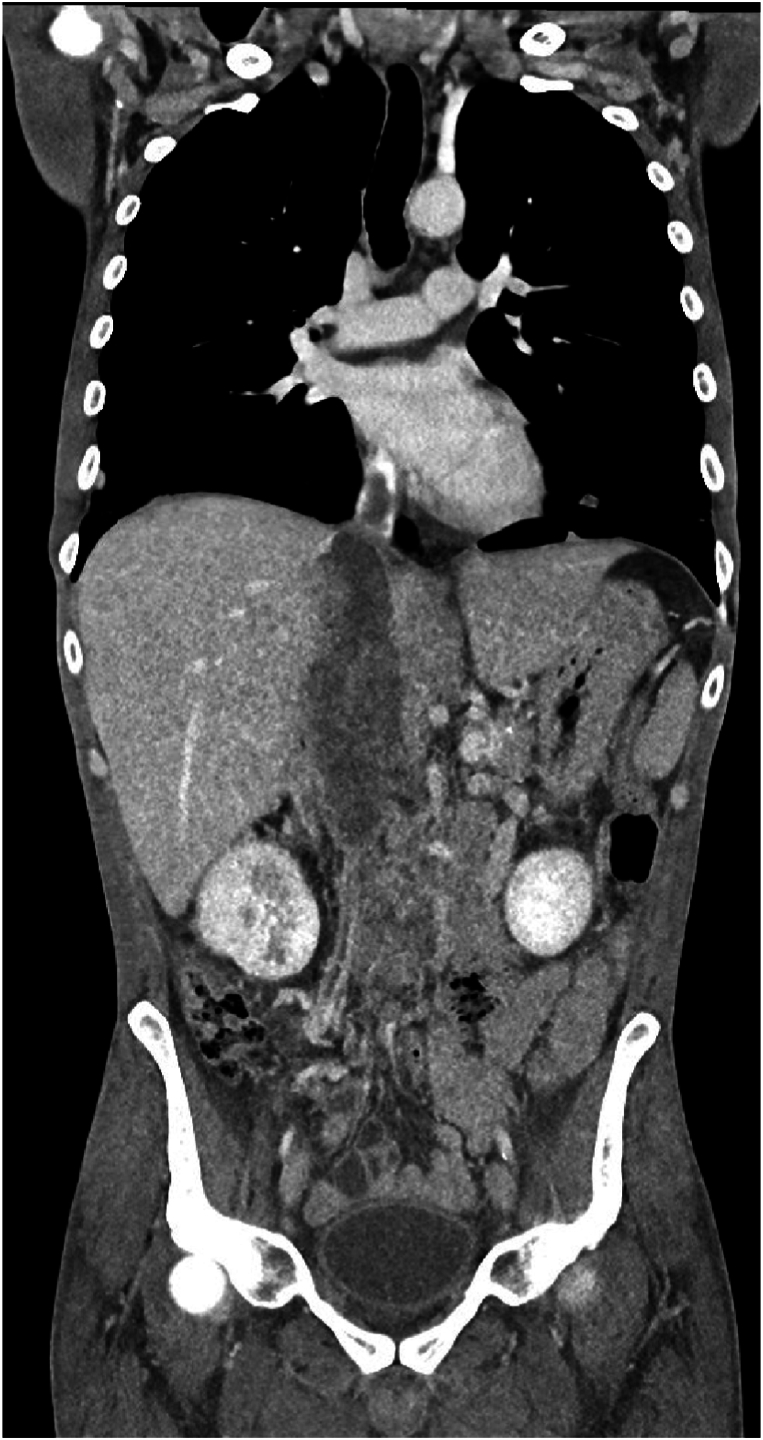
A contrast-enhanced computed tomography scan of the abdomen and thorax: A large tumorous formation of the right adrenal gland, with thrombus extending into the IVC and right atrium.
